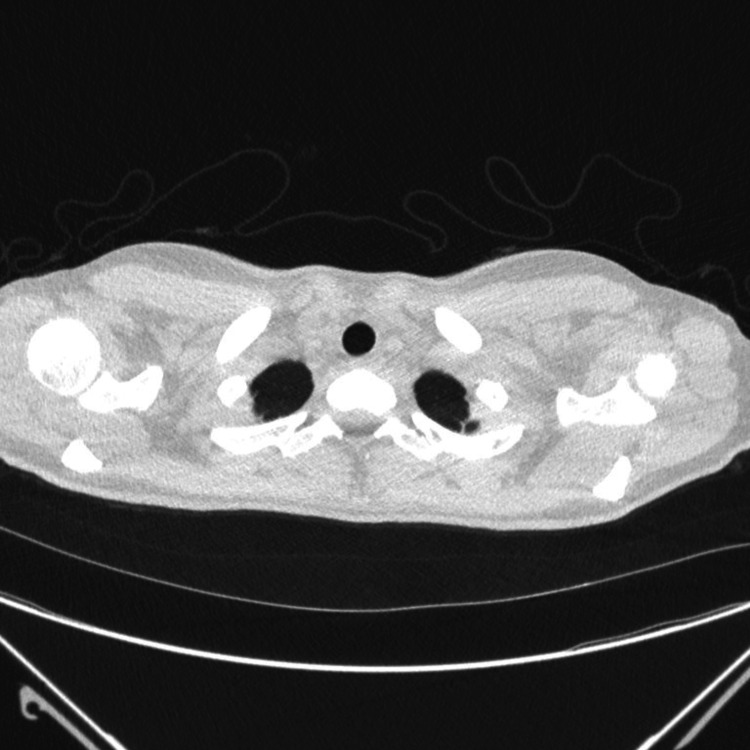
Axial CT chest at outpatient follow-up The scan demonstrates complete resolution of the previously noted subcutaneous emphysema and pneumomediastinum.
